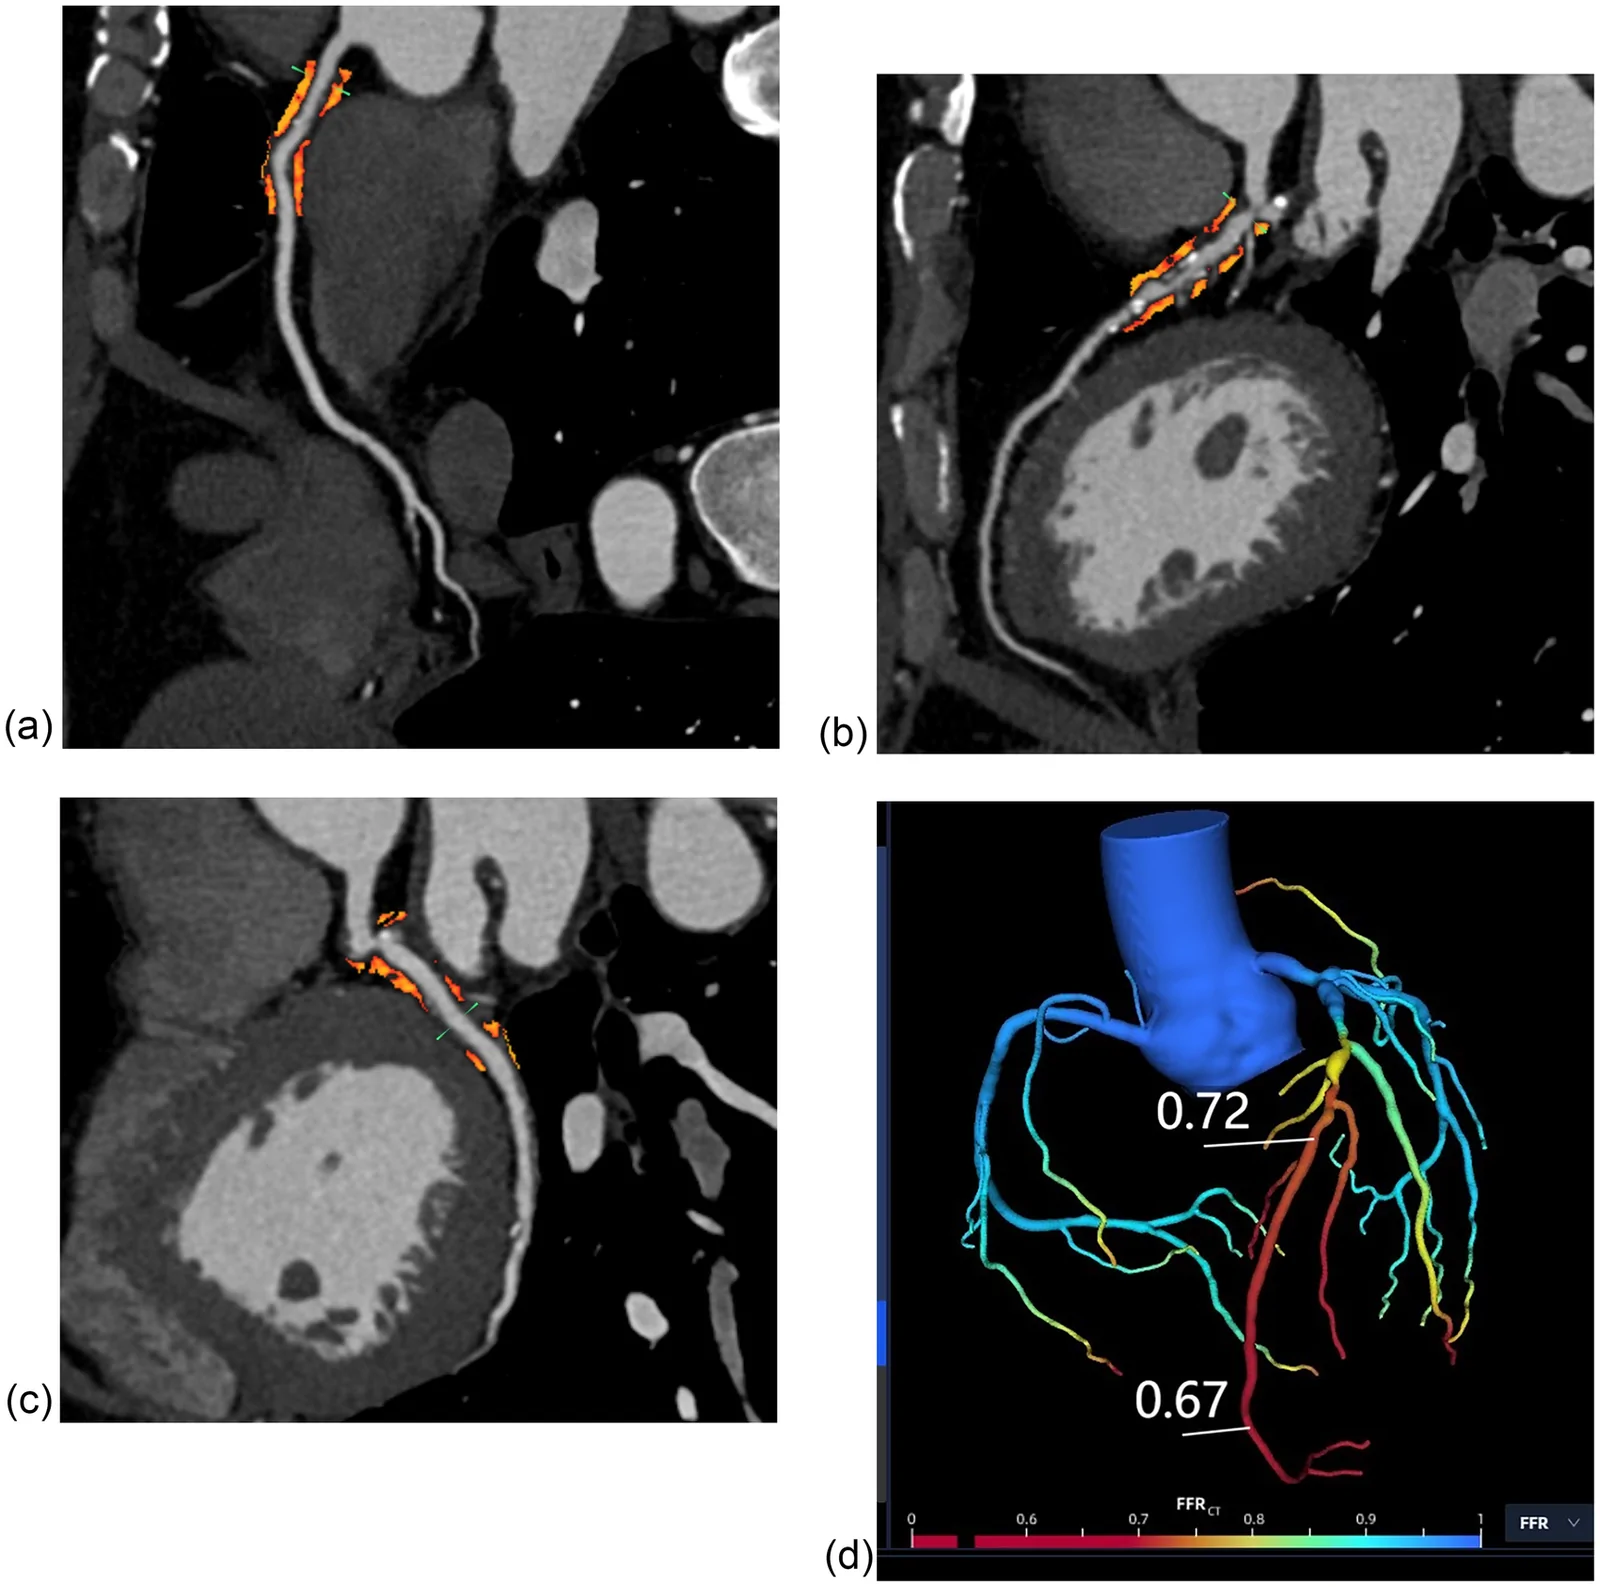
Representative imaging findings from a 48-year-old male patient classified as high risk by the RF-CL model. (a) RCA-FAI measurement (−79.90 HU) with the peri-coronary adipose tissue shaded in color; (b) LAD-FAI (−91.20 HU); (c) LCX-FAI (−83.03 HU); (d) CT-FFR color-coded map showing plaque-distal CT-FFR of 0.72 and distal vessel CT-FFR of 0.67. CACS was 476.5 and CAD-RADS grade 3. FAI, fat attenuation index; RCA, right coronary artery; LAD, left anterior descending; LCX, left circumflex; CT-FFR, computed tomography fractional flow reserve; CACS, coronary artery calcium score.
